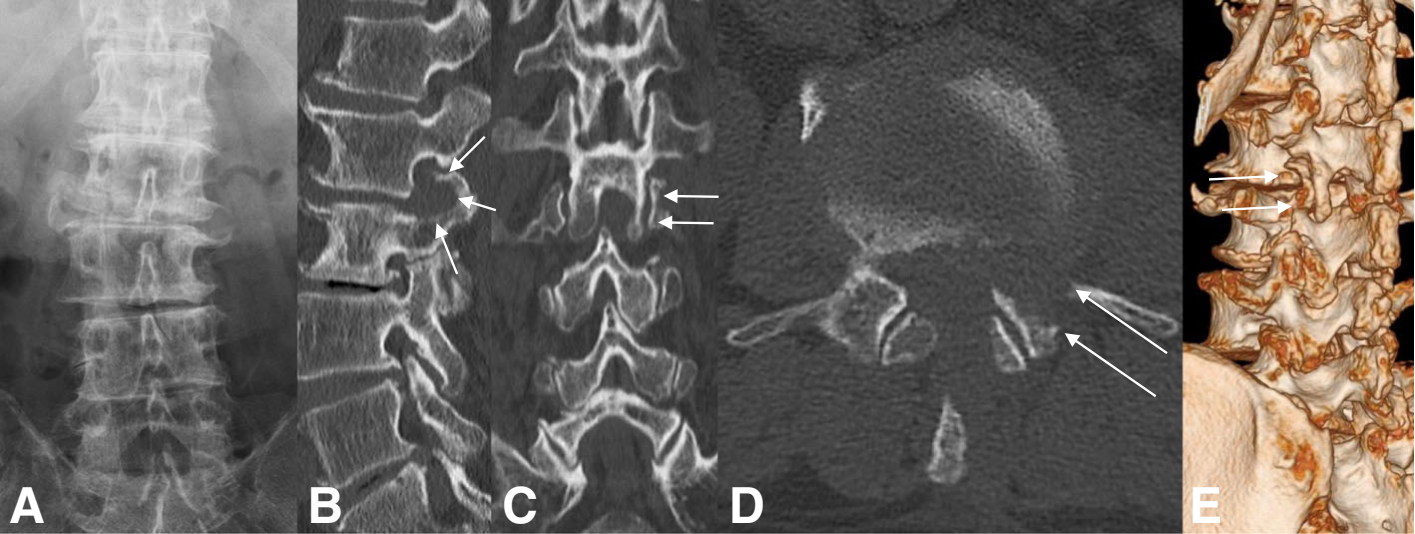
Postoperative X-ray and computed tomography demonstrated the complete preservation of the articular surface of the facet joint. a X-ray; b sagittal view; c coronal view; d axial view; and e three-dimensional computed tomography – oblique view. The *arrows* are pointing to the the complete preservation of the articular surface of the facet joint and the trajectory to the L2-3 extraforaminal space
